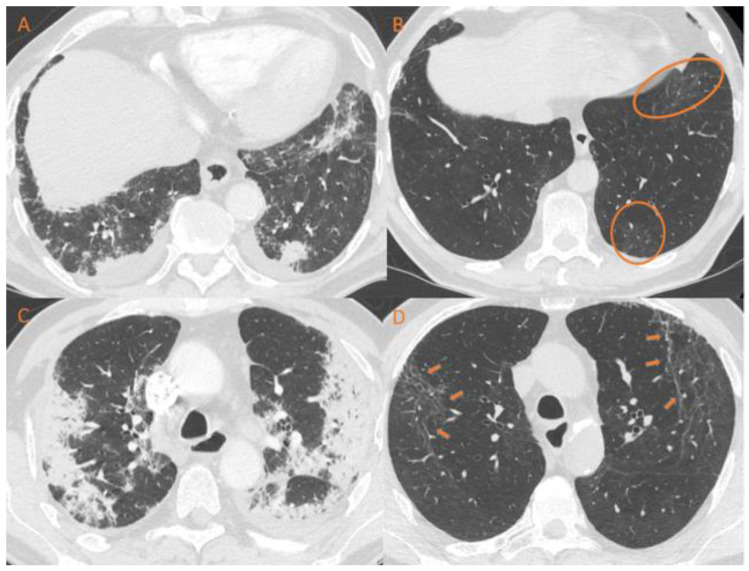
Example of CT-scan evolution in a 76-year-old patient. (A,C) acute COVID-19 phase. (B,D) CT-scan performed 7 months later showing persistent ground glass opacities (B) and retractile reticulations (D).
